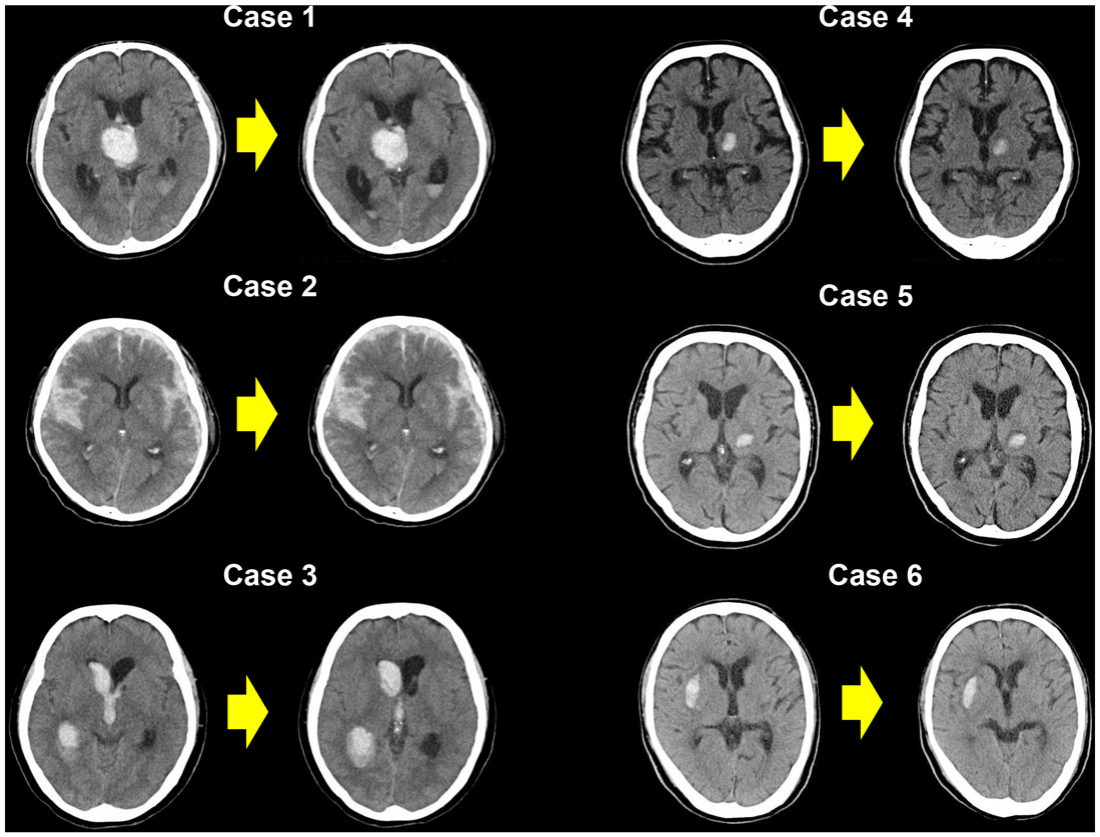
Brain computed tomography (CT) on admission (left) and 24 h later (right) in Cases 1, 3–6, 1.5 h later (right) in Case 2 who had intracranial hemorrhage during NOAC therapy. No hematoma expansion is evident in any of the cases.
